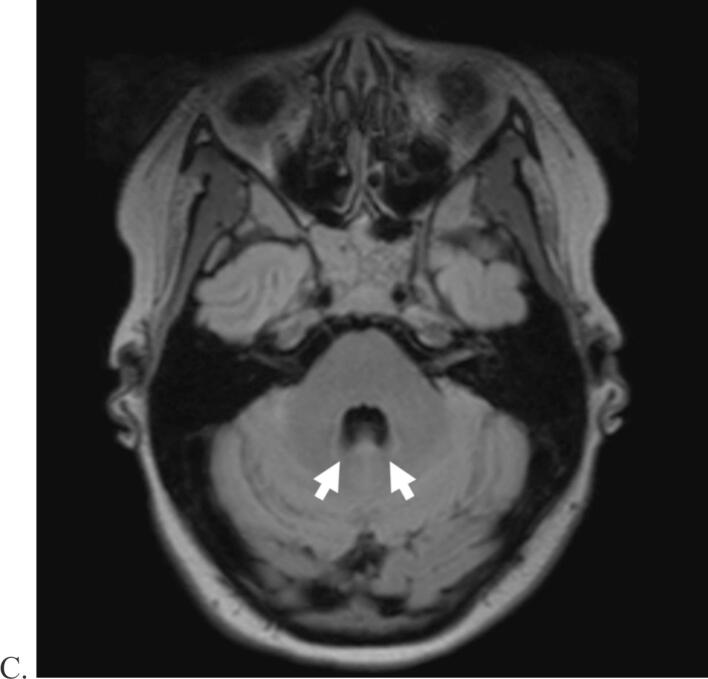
Magnetic resonance imaging of our patient. A) Coronal T2-weighted sequence showing partial midline fusion of the cerebellar hemispheres with continuity of the folia (white arrow). B) Sagittal T2-weighted flair sequence showing subtle incomplete development of vermis. C). Axial T2-weighted flair sequence showing slight medialization of the dentate nuclei (white arrows).
